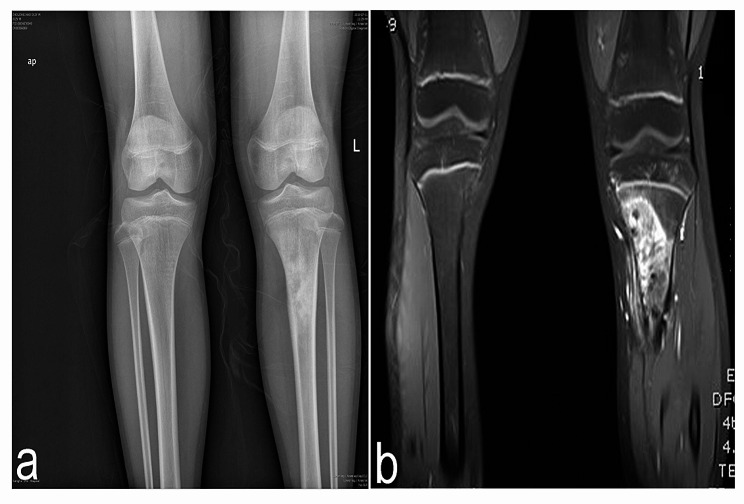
Pictures of Patient. X-ray radiograph (a) and magnetic resonance I maging (MRI) (b) of the proximal tibia, showing a lesion that did not affect the proximal epiphyseal
